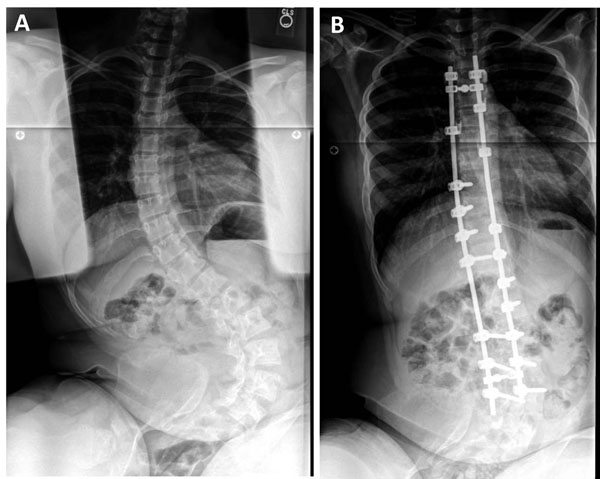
Surgical management of progressive scoliosis in a patient with fibrous dysplasia. Pre-treatment radiograph shows extensive scoliosis with both a thoracic and lumbar curve (A). The same patient is shown after posterior spinal fusion (B).
